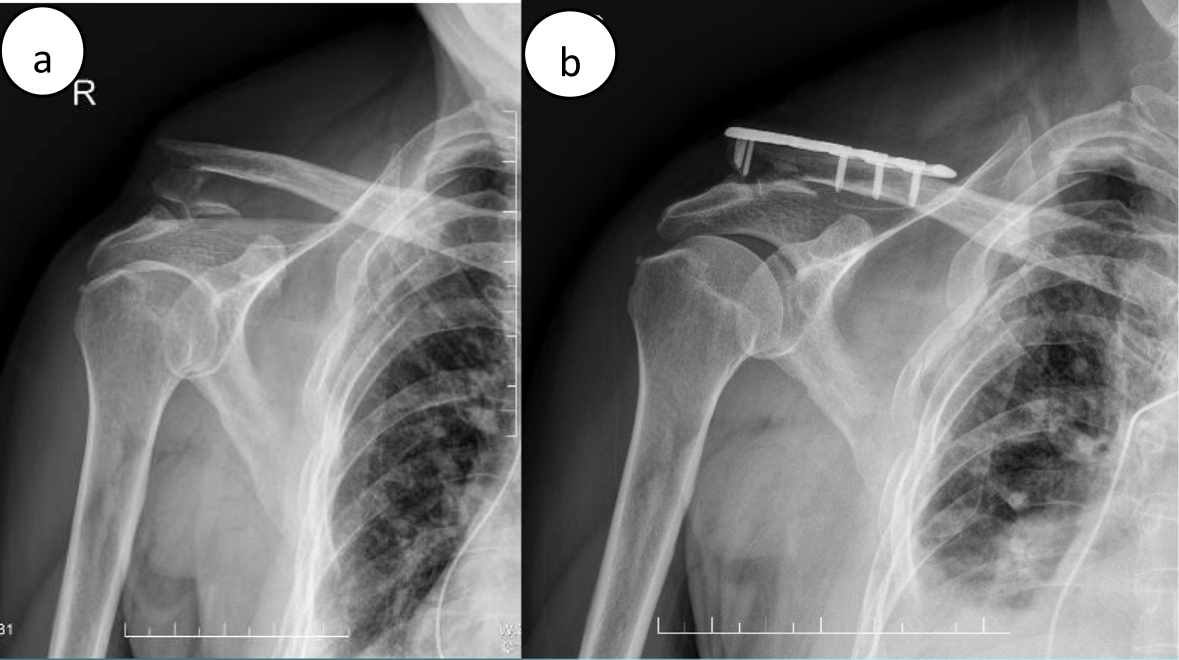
a . X-ray of a patient before surgery. b. Post-operation x-ray showed loss of reduction
